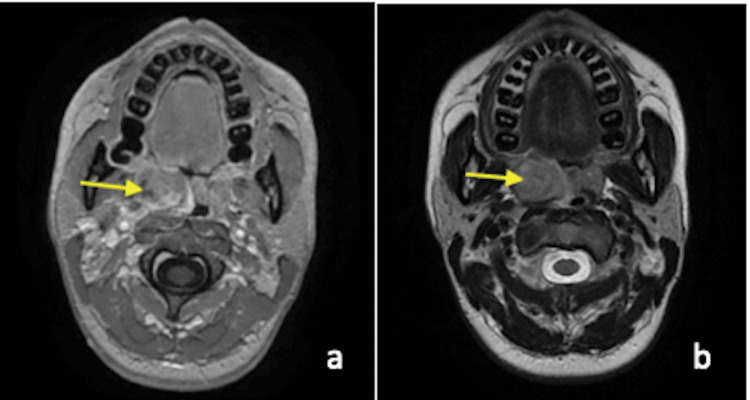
MRI in axial sections, the yellow-arrows show unilateral swelling of the right tonsil with areas of necrosis a: T1 with gadolinium; b: T2
